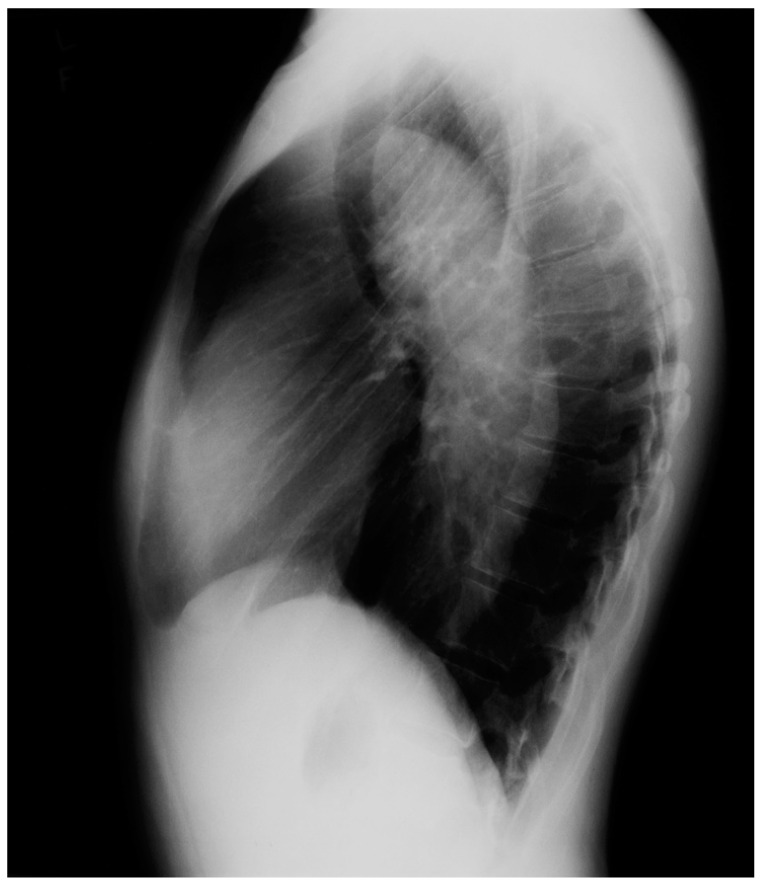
Lateral chest X-ray of Marfan syndrome: this chest X-ray was done on the same 24-year-old female above. It shows the dorsal kyphosis and pectus carinatum (also known as pigeon chest). The ascending, arch, and descending aorta were markedly dilated. Additional imaging measured the minimum thoracic aortic diameter to be 6 cm. She went on to have a mechanical aortic valve replacement, followed by aortic root and ascending aortic replacement via median sternotomy, and then descending aortic replacement via left lateral thoracotomy. A few years later she required an extensive abdominal aortic replacement with multiple arterial anastomoses to vital organs supplied by the abdominal aorta. She was followed for 30 years after her first diagnosis of MFS and her first aortic operation and remained NYHA functional class 1–2. Suspicion of the diagnosis of MFS in an asymptomatic patient that leads to a CXR is an important first step in the optimal care of MFS.
